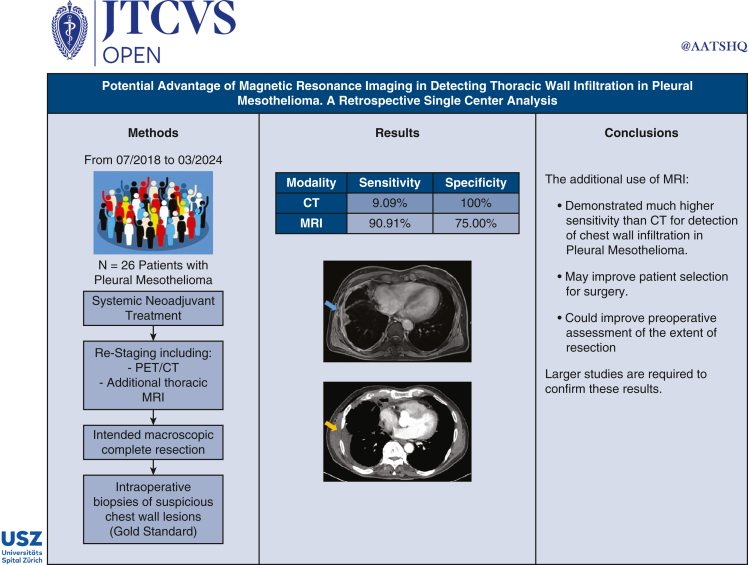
Graphical Abstract: Single-center retrospective analysis of the potential benefit of additional thoracic MRI for assessing chest wall infiltration during pleural mesothelioma restaging after neoadjuvant systemic therapy and before resection. *PET*, Positron emission tomography; *CT*, computed tomography; *MRI*, magnetic resonance imaging.
